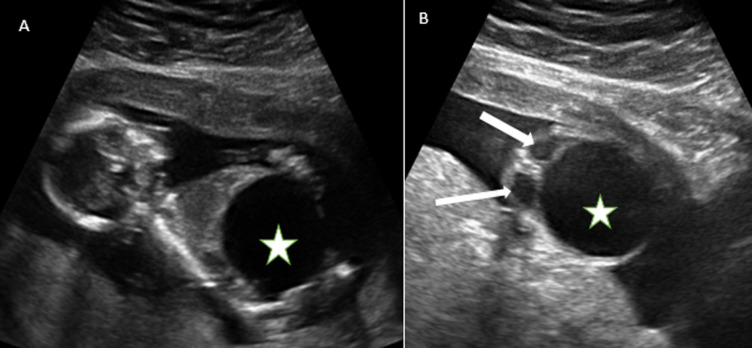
sagittal (A) and transverse (B) ultrasound scan images of a fetus at 18 week gestational age showing gross distended urinary bladder (star) consistent with megacystis - a severe fetal anomaly and dilated renal pelves bilaterally (arrows)
